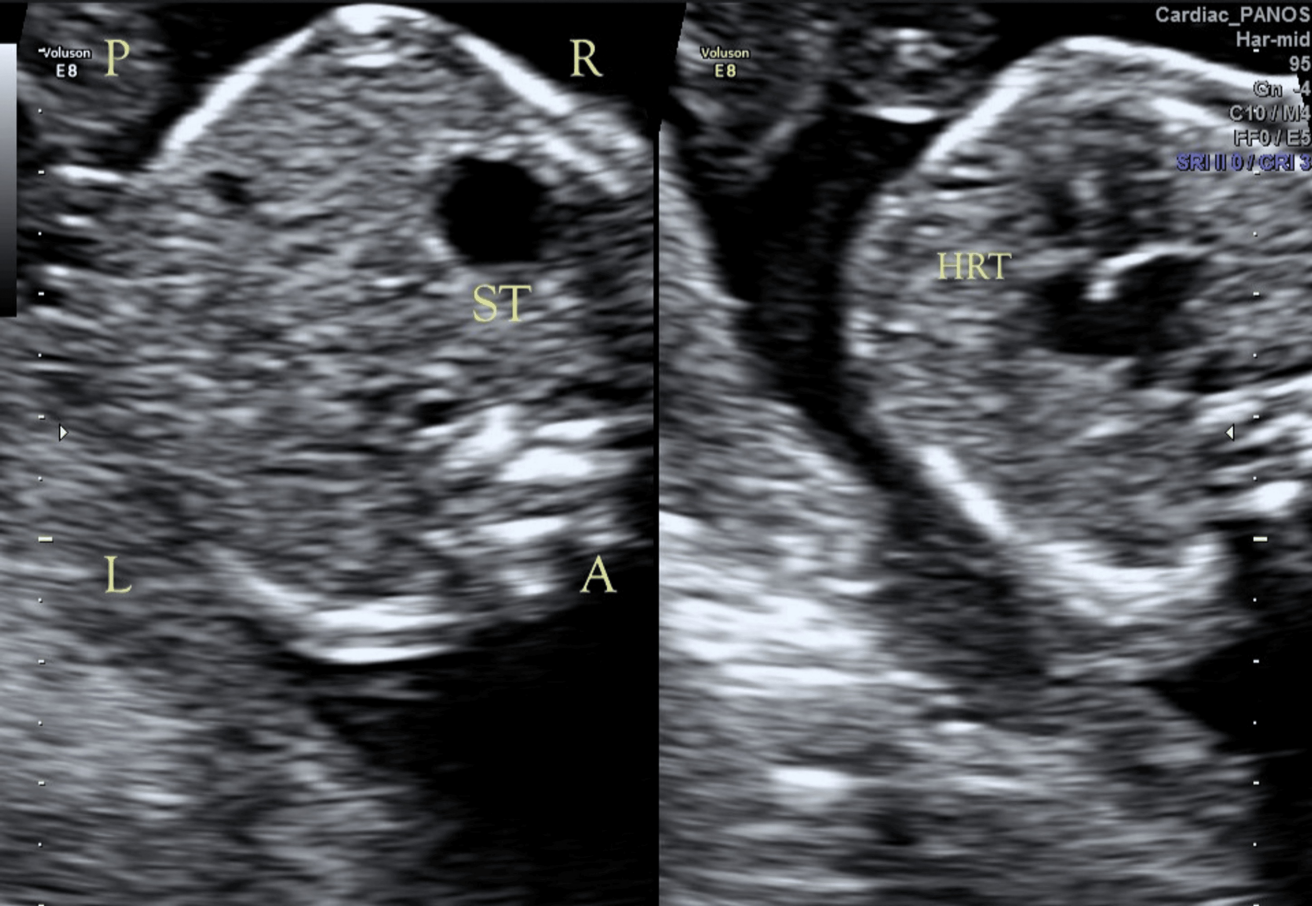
Transverse ultrasound images Transverse ultrasound images of the fetal abdomen (left) and thorax (right) obtained at 20 weeks and four days gestation. Orientation markers indicate fetal right (R), left (L), anterior (A), and posterior (P). The fetal stomach (ST) is visualized on the right side of the abdomen, and the fetal heart (HRT) is visualized with its apex directed toward the right thorax. These findings are suggestive of a right-sided visceral and cardiac orientation (right-sided stomach and heart).
